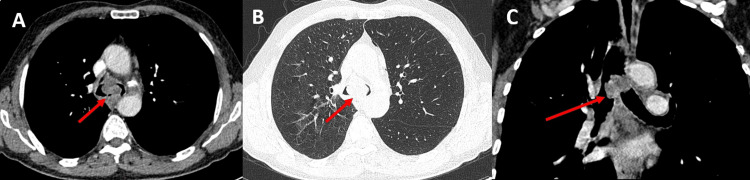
Thoracic CT scan showing tissue thickening (red arrows) that invaded the carina and protruded into the endobronchial region. A: axial section with mediastinal window, B: axial section with parenchymal window, C: coronal section with mediastinal window.
